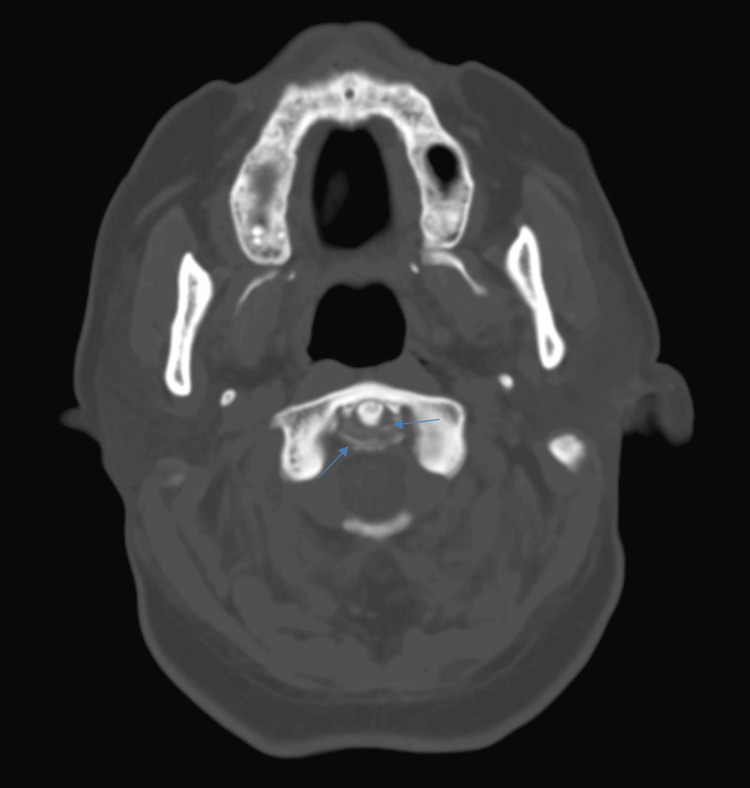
Axial CT scan of the cervical spine CT scan of the patient's cervical spine demonstrated calcifications around the odontoid process (dens) of C2, consistent with crowned dens syndrome. The calcifications are visualized encircling the dens, forming a crown-like structure in the transverse retro-odontoid ligament. This imaging finding is characteristic of calcium pyrophosphate deposition (CPPD) and correlates with the patient’s clinical presentation of neck pain, fever, and elevated inflammatory markers.
